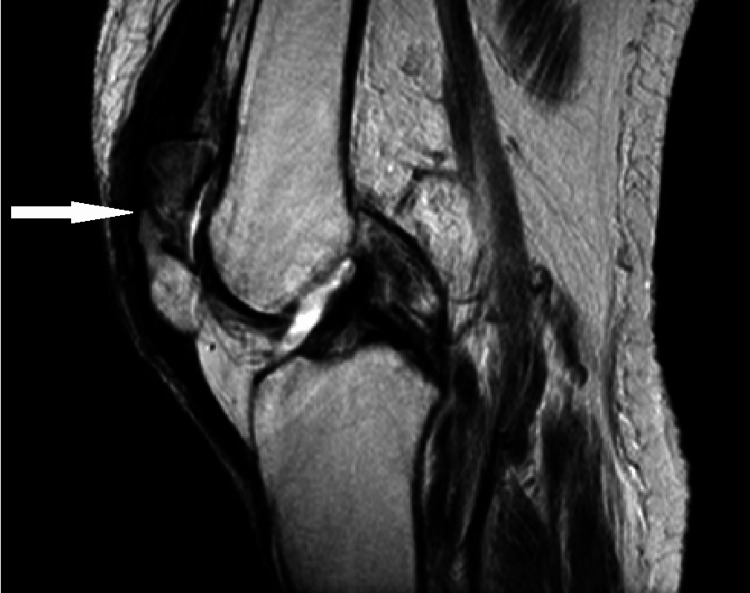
Sagittal view non-contrast T1-weighted MRI of the patient's right knee showing patellar deformity consistent with comminuted fracture shown in Figure 1. No evidence of fusion of the fracture is consistent with nonunion. Small knee effusion with generalized edema of the subcutaneous soft tissues was also seen.
